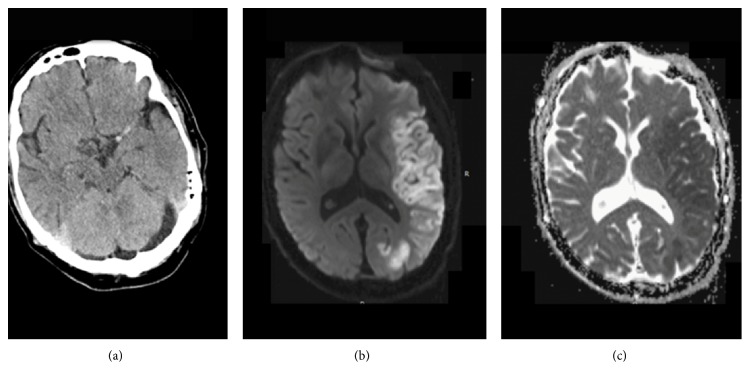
Hyperdense middle cerebral artery on the initial CT prior to intravenous tissue plasminogen activator (a). Diffusion-weighted magnetic resonance imaging (b). Apparent diffusion coefficient (c) confirms acute infarction in the left MCA territory.
